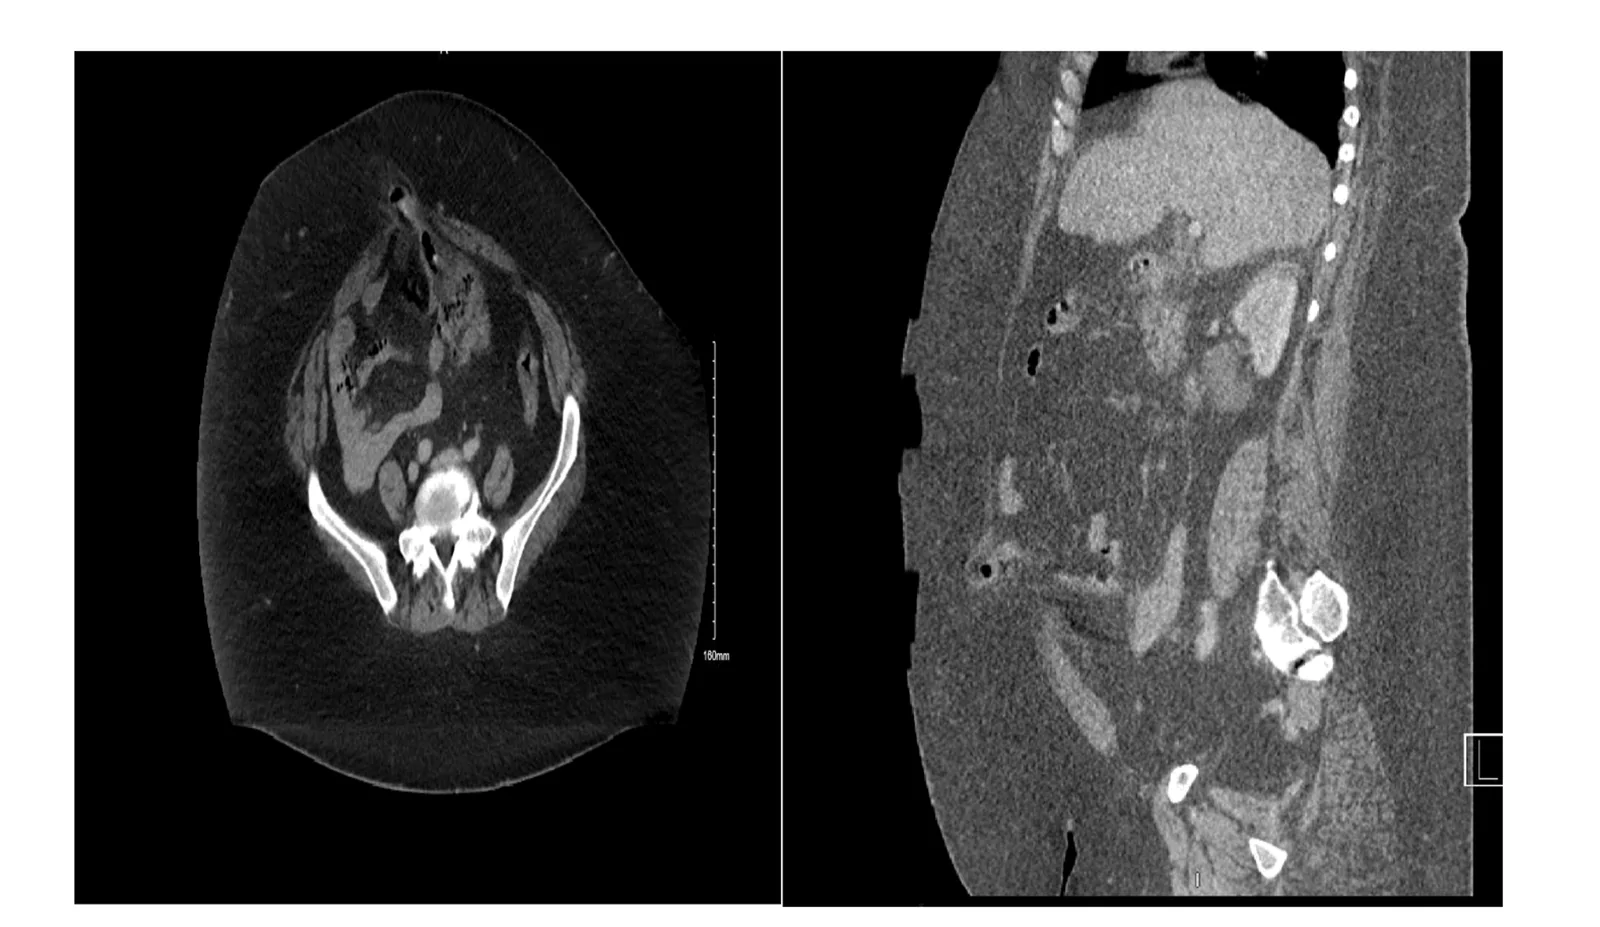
CT axial and sagittal sections showing paraumbilical hernia defect with its content
